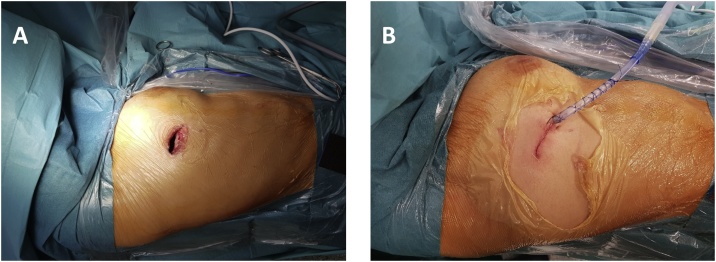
Wound before (A) and after suture (B). Picture B shows the placement of a transthoracic drainage.
